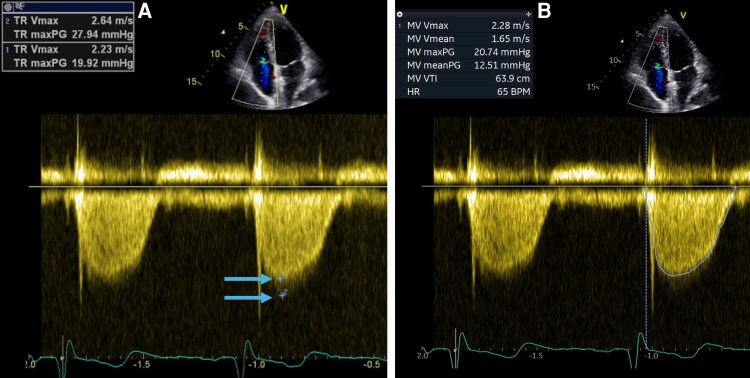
Assessment of Doppler velocities at the outer modal velocity spectrum. TR velocity at the top of the fringes shows a Vmax of 2.64 m/sec, whereas a significant lower Vmax of 2.23 m/sec is obtained when measuring at the outer edge of the intense modal velocity (*A*). Tracing of the TR signal to obtain the VTI also occurs at the outer edge of the modal velocity, thus excluding the fringes (*B*).
